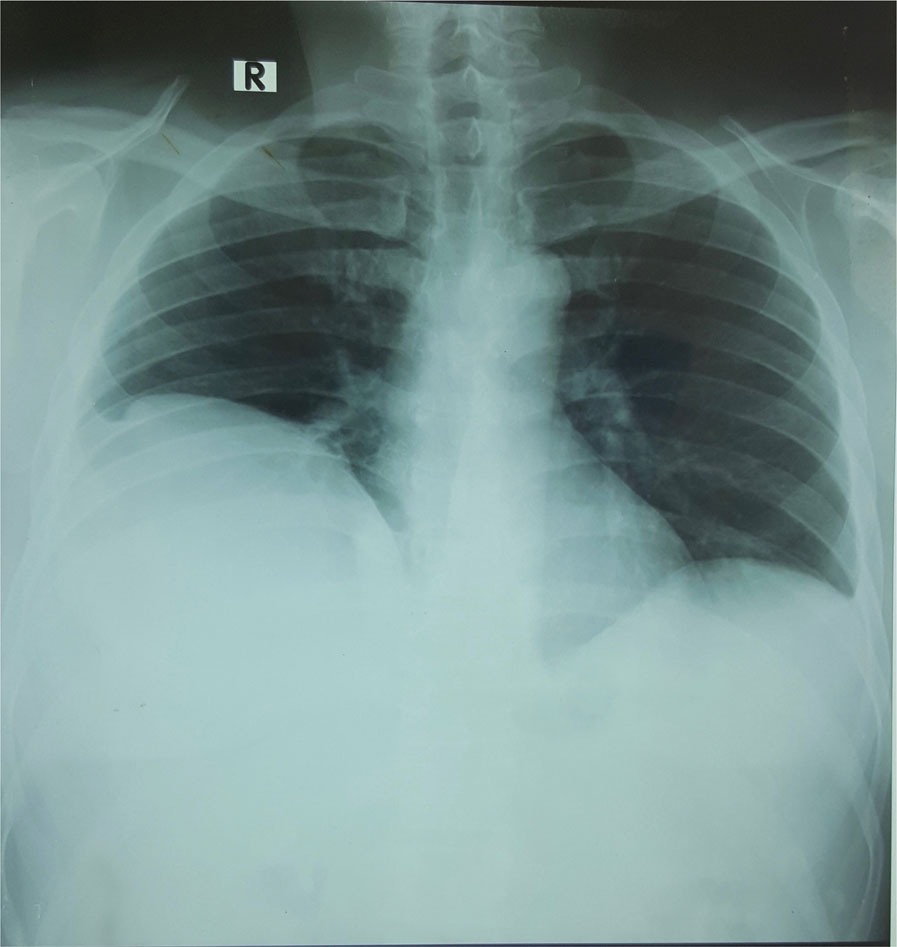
A posteroanterior chest X-ray showed right-side hemidiaphragm elevation
